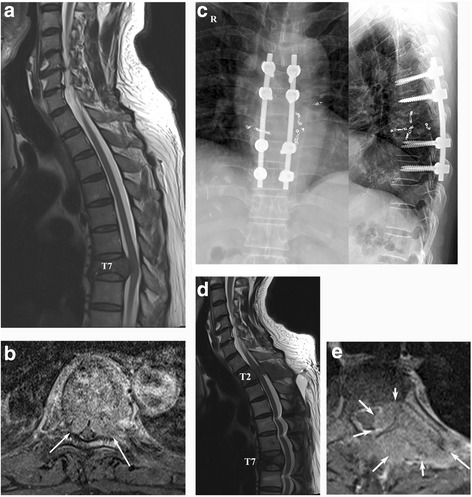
A 54-year old man with HCC who developed MSCC. a Preoperative T2-weighted sagittal MR image showing cord compression at *T7*. b Preoperative T1-weighted enhanced MR image (*arrows* indicate cord compression by the tumor mass). c Postoperative radiographs. d T2-weighted sagittal MR image 6 months postoperatively showing another occurrence of cord compression at *T2*, with maintenance of the decompression at *T7*. e T1-weighted enhanced MR image at *T2* (*arrows* indicate tumor mass). Hepatocellular carcinoma (HCC), metastatic spinal cord compression (MSCC)
